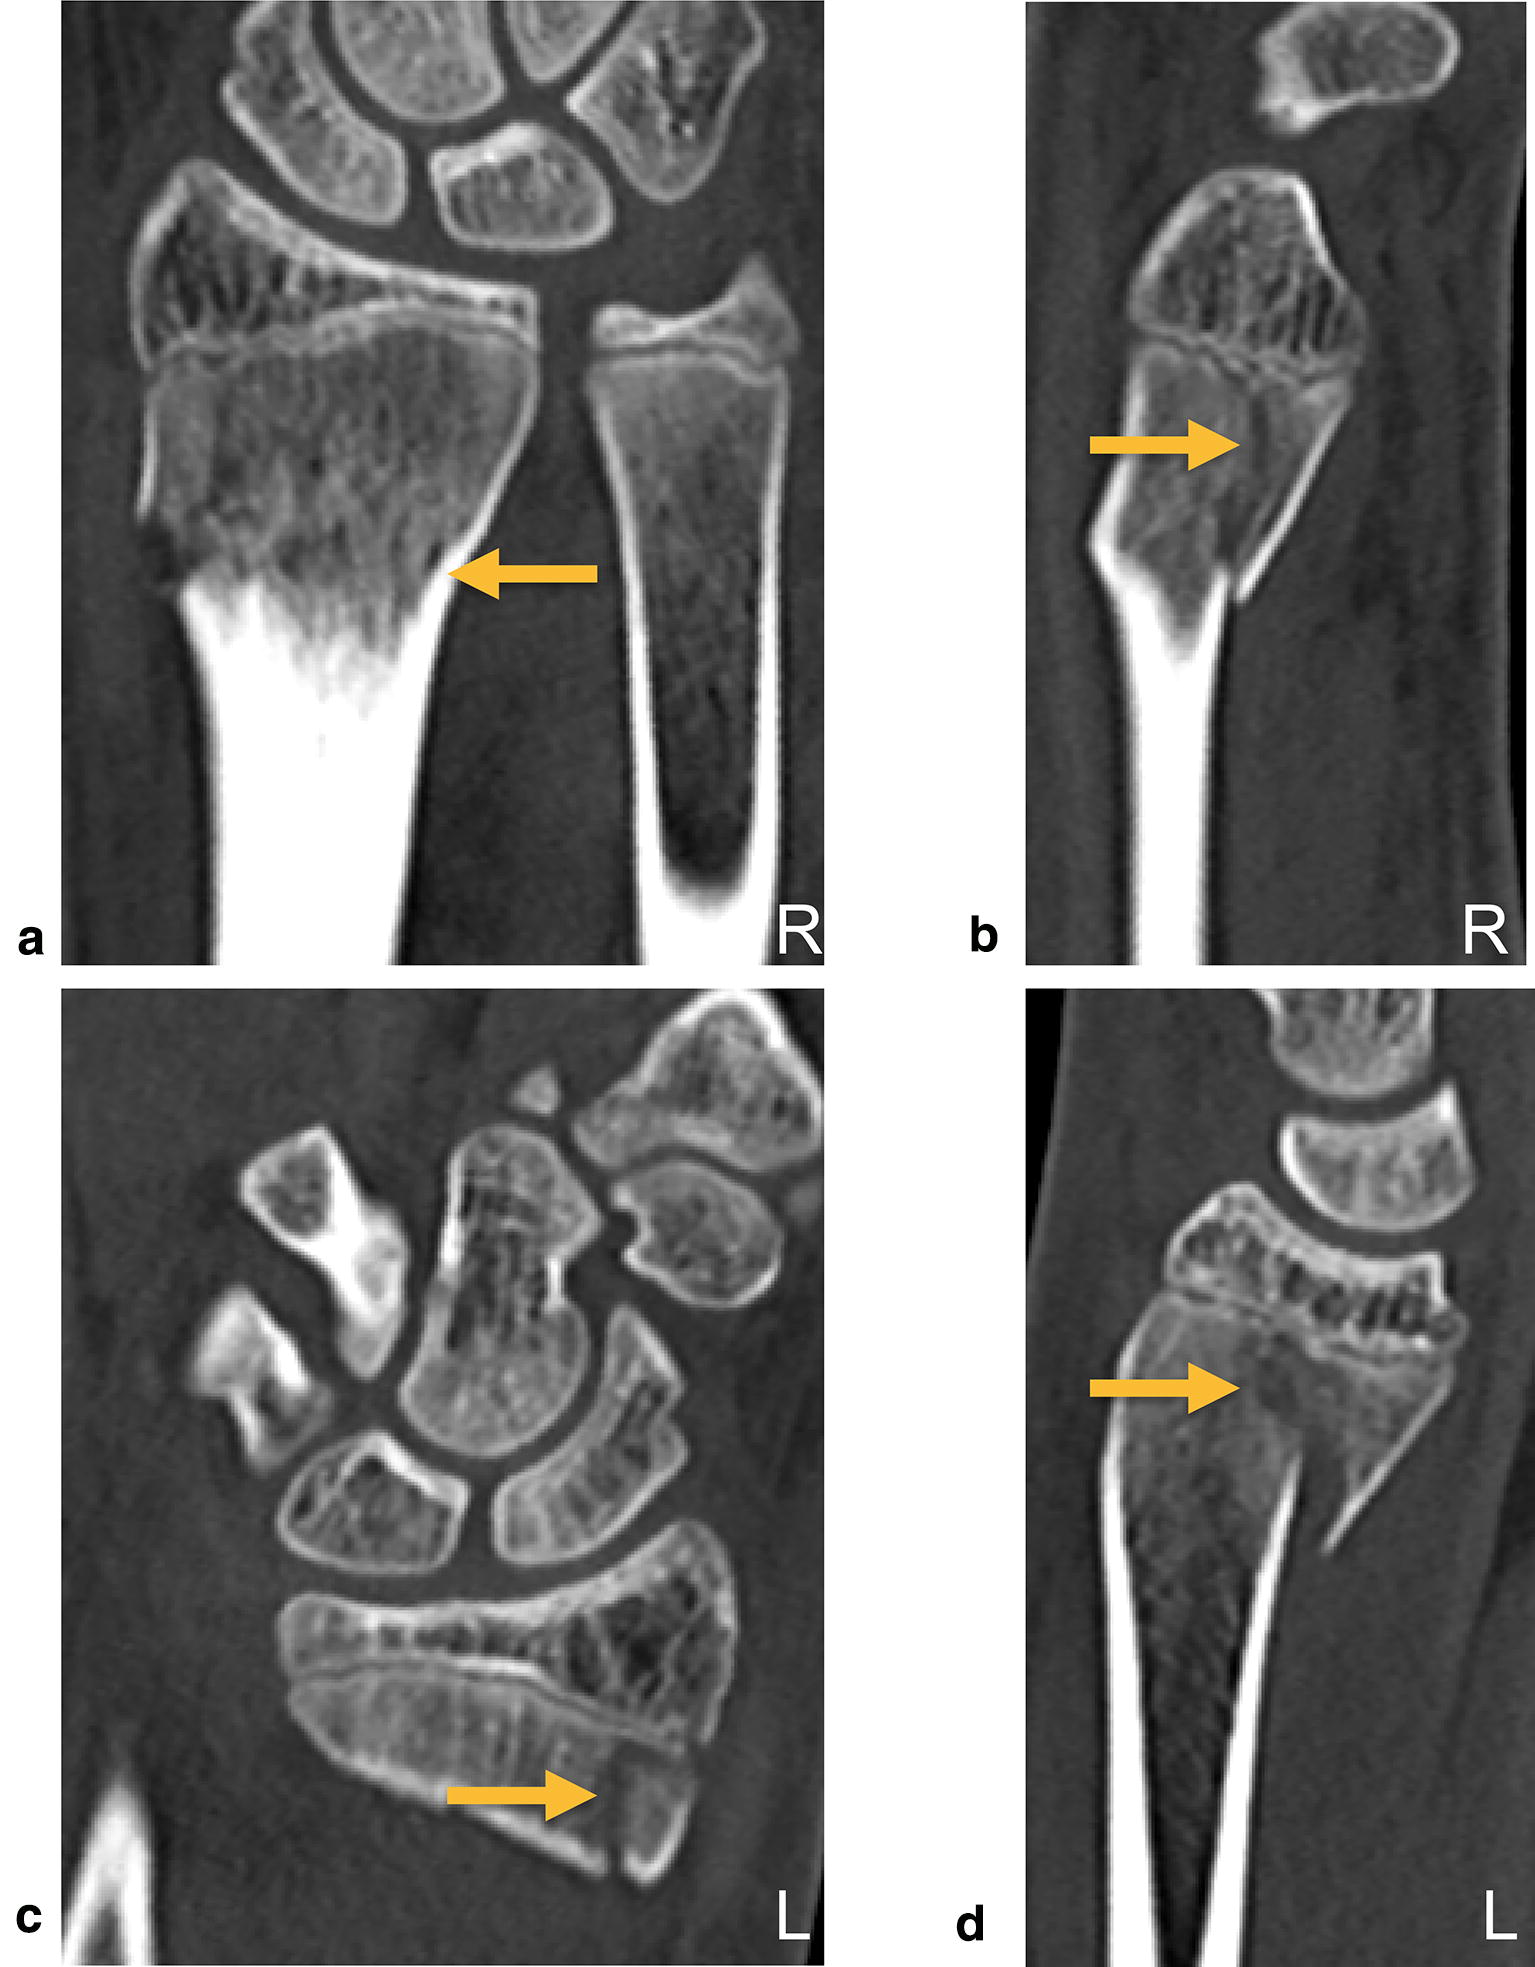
Diagnostic imaging case 2, CT scan (a coronal view right side, b sagittal view right side, c coronal view left side, d sagittal view left side) demonstrating a displaced transitional fracture of the distal radius on both sides (arrows)
